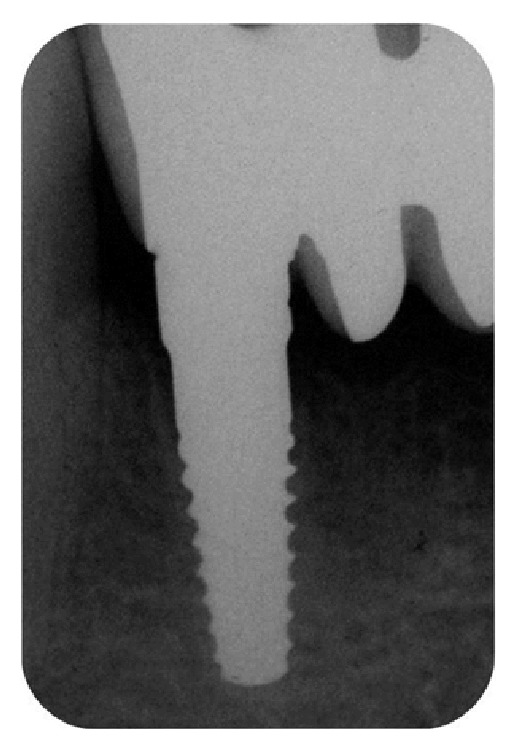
X-ray image of the zirconia implant placed in area 4.2, six months after surgery.
